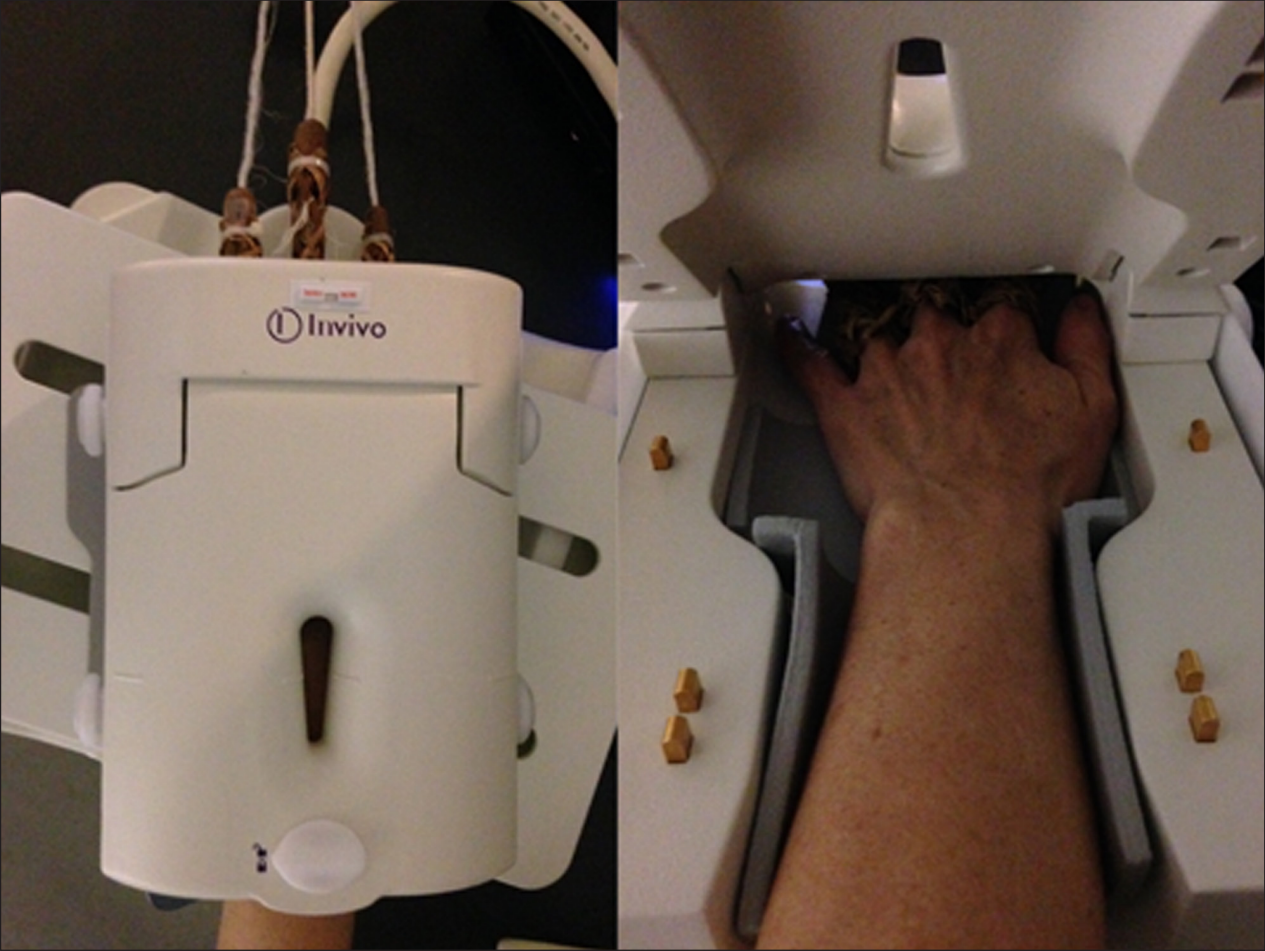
Outside and inside view of the wrist during wrist MRI in traction.
